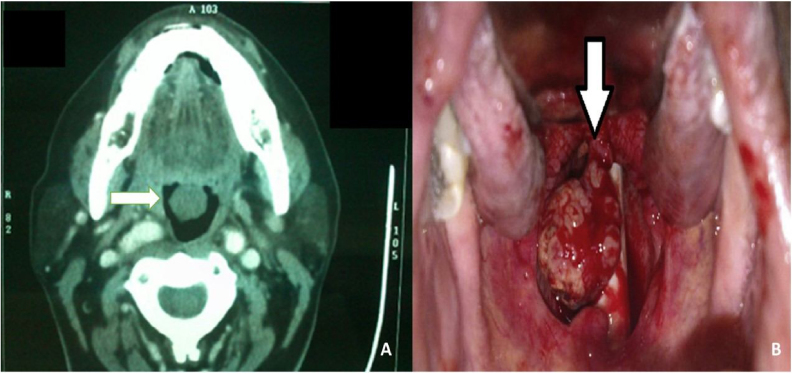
(A) Image showing the axial section of contrast CT scan of Base of Tongue Schwannoma. The white arrow pointing towards the right side shows the schwannoma with stalk attached to the base of the tongue. (B) An intraoperative picture of schwannoma arising from the base of the tongue is shown by the white arrow pointing downwards.
